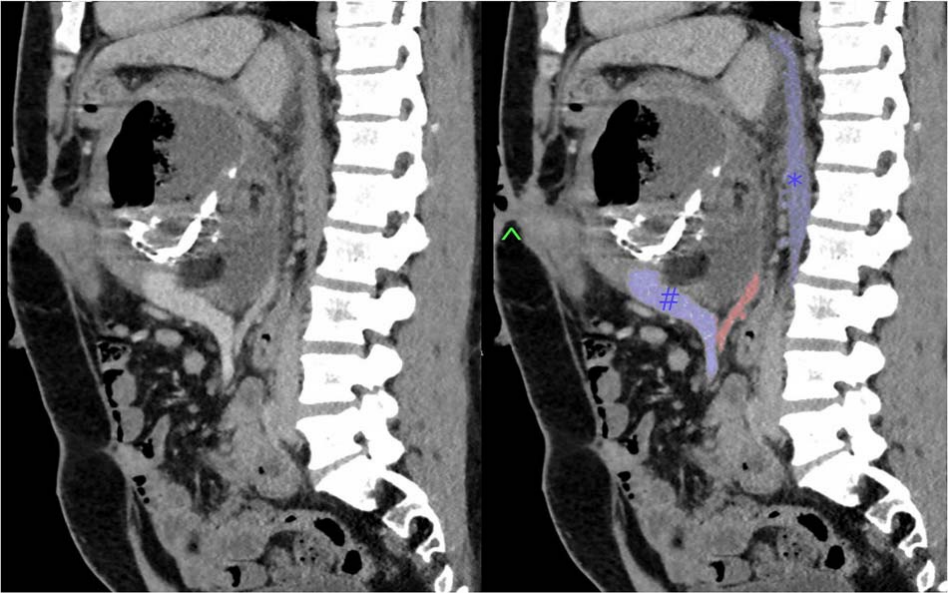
Sagittal section of pre-operative CT with portal venous contrast showing the lesions relationship to major vascular structures of the abdomen. Labels: ^ - fistula opening, blue # - superior mesenteric vein, blue * - inferior vena cava, red - superior mesenteric artery.
